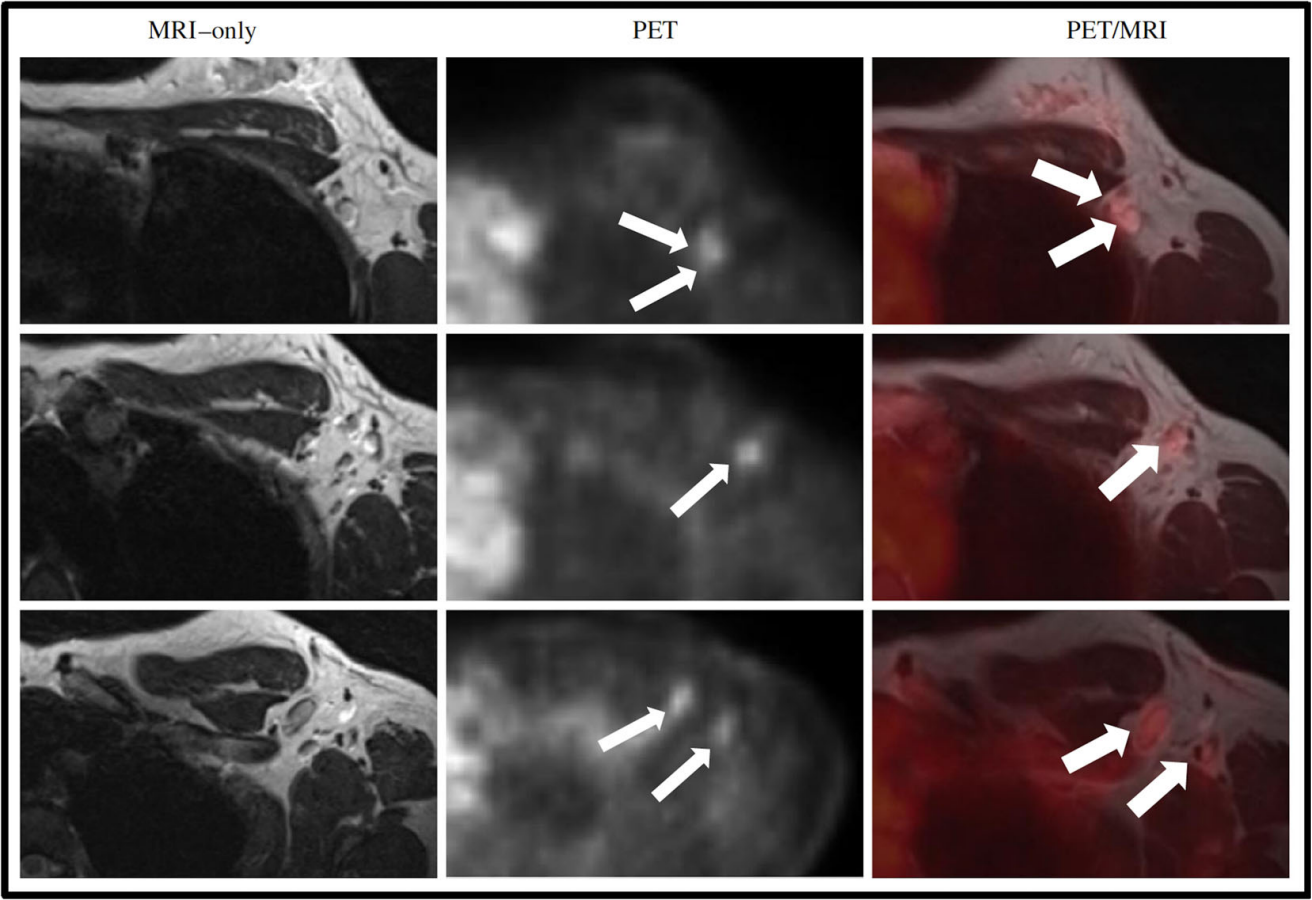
Images of a patient with no lymph nodes suspicious for metastases on MRI (T2w sequence is shown in the left column) and five axillary FDG hotspots suspicious for lymph node metastases on PET (small arrows, middle column). Adding PET information to MRI, resulted in five lymph nodes marked as suspicious for metastases (big arrows, right column). Reprinted under a Creative Commons (CC BY 4.0) license from: Goorts B, Vöö S, van Nijnatten TJA, Kooreman LFS, de Boer M, Keymeulen KBMI, Aarnoutse R, Wildberger JE, Mottaghy FM, Lobbes MBI, Smidt ML. Hybrid ^18^F‐FDG PET/MRI might improve locoregional staging of breast cancer patients prior to neoadjuvant chemotherapy. Eur J Nucl Med Mol Imaging. 2017;44(11):1796–1805.
